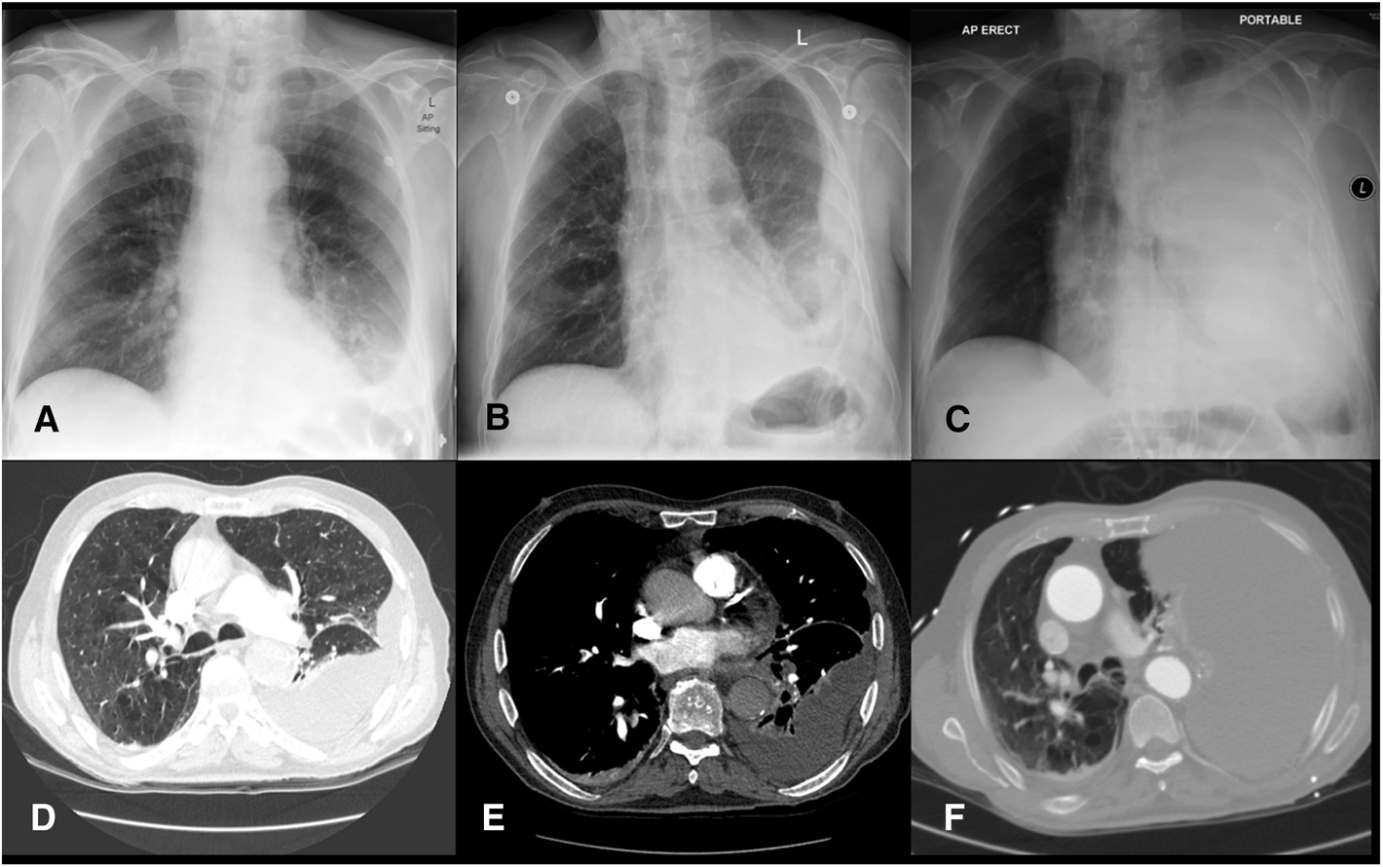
a Chest radiograph on presentation at hospital. b Chest radiograph after initial pigtail drain insertion. c Portable chest radiograph during medical emergency call. d Transverse contrast-enhanced computed tomography image on presentation to hospital – lung window. e Pulmonary embolus in division of right pulmonary artery. f Computed tomography angiogram following medical emergency team call
